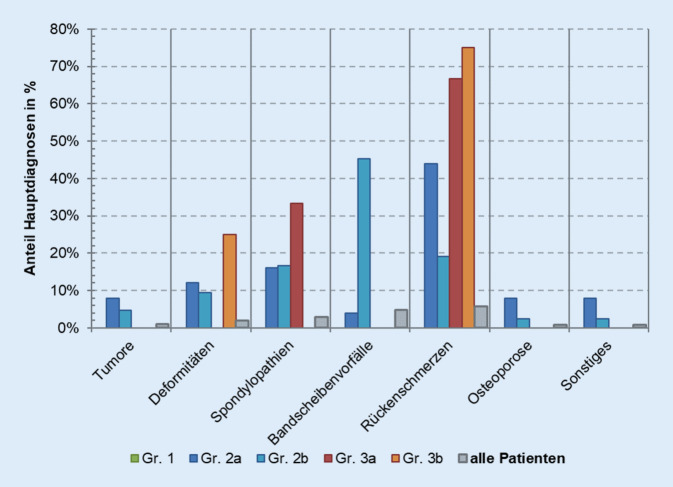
Eine weitere Differenzierung der Gruppen erfolgte nach dem diagnostischen Aufwand. 65,9 % der Patienten erhalten eine Röntgenuntersuchung eines spezifischen Abschnittes der Wirbelsäule beim ersten Aufenthalt. 30 % der Patienten erhielten im Rahmen des ersten stationären Aufenthaltes eine kernspintomographische Untersuchung der Wirbelsäule. Dabei bestand keine Signifikanz zwischen den einzelnen Gruppen. Weitere schnittbildgebende Verfahren wie die CT oder PET-CT spielten keine wesentliche Rolle (Abb. 7). Eine Messung der Knochendichte wurde in 15 % der Fälle durchgeführt. Bei den weiteren Aufenthalten sinkt der diagnostische Aufwand, da die Patienten in der Klinik bekannt sind (Abb. 8).
